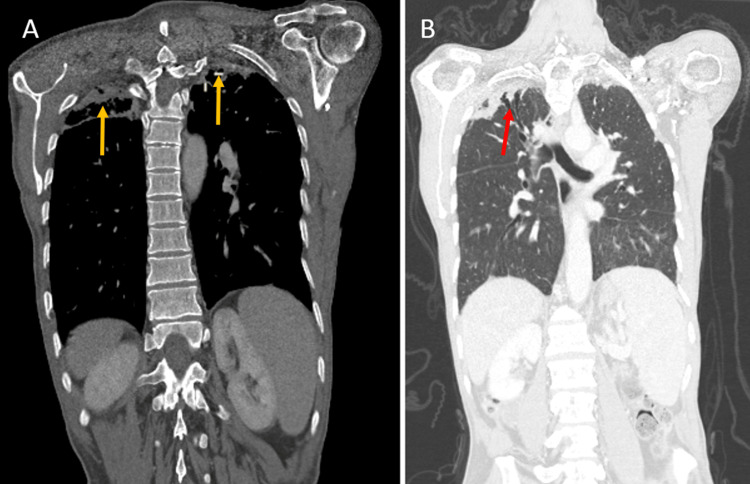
A: Axial view thoracic computed tomography showing apical pleural thickening and subpleural consolidations (yellow arrows). B: Axial view thoracic computed tomography showing traction bronchiectasis (red arrow).
